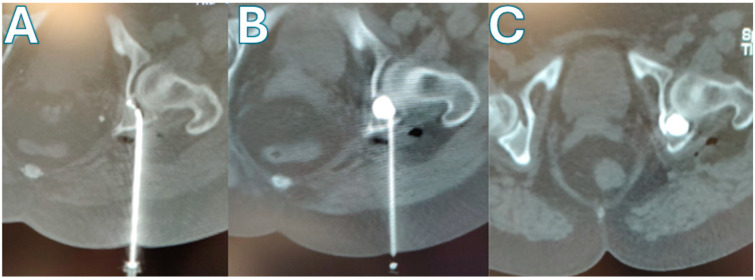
CT/fluoroscopic-guided Balloon-Assisted Acetabuloplasty in a 53-year-old patient with metastatic breast carcinoma involving the left posterior acetabulum. (A) Axial CT image showing percutaneous placement of a curved radiofrequency ablation probe within the osteolytic acetabular lesion. (B) Balloon-assisted cavity creation using a curved balloon catheter; contrast opacification confirms adequate expansion and cavity formation within the ablated lesion. (C) Post-procedural CT demonstrating satisfactory PMMA cement filling of the lesion with appropriate distribution and no evidence of intra-articular leakage.
